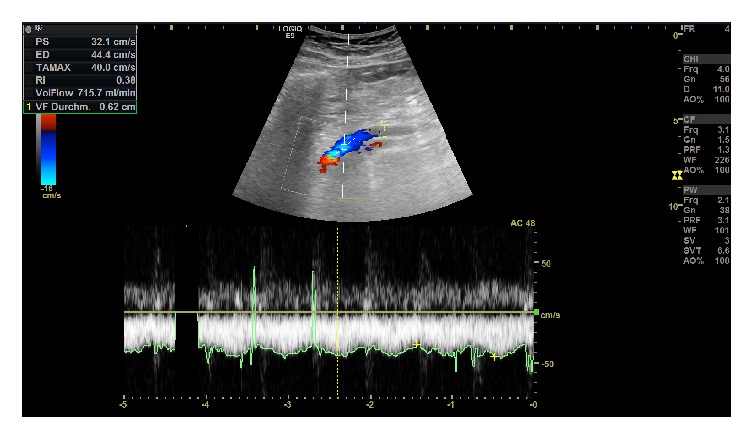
Duplex ultrasonography examination to verify unimpaired portal blood flow in the postoperative course.
